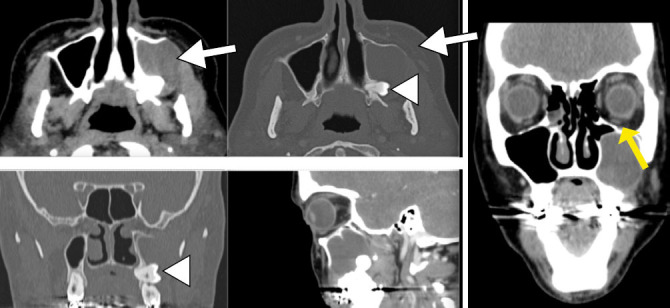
Dentigerous cyst in a 44-year-old female patient. CT scans showing a
